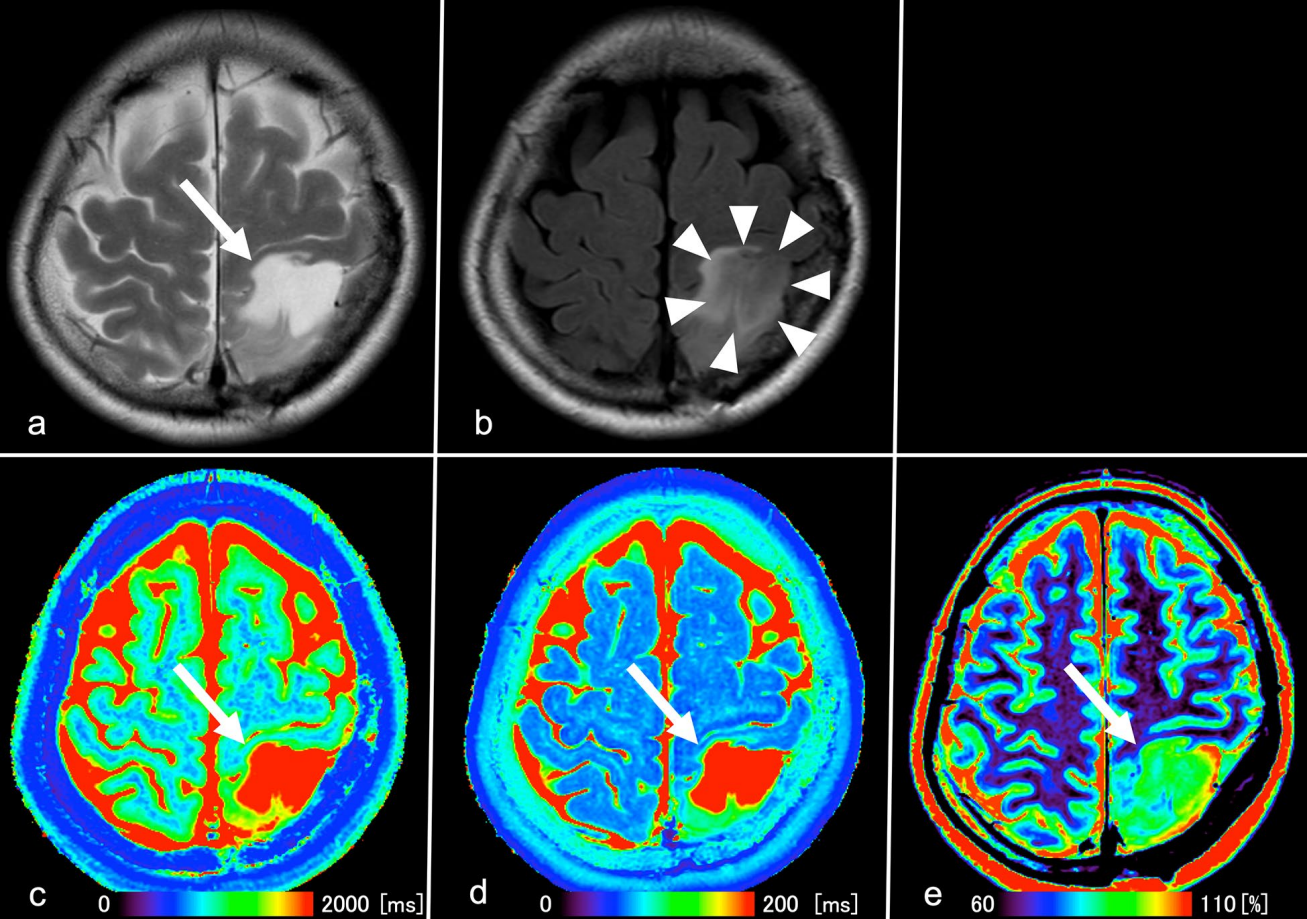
Images from a 49-year-old woman with IDH-mutant diffuse astrocytoma (WHO grade II). (a) T2WI shows a heterogeneous T2-prolonged mass in the left parietal lobe (arrow). (b) FLAIR shows partial signal suppression, indicating a T2-FLAIR mismatch sign (arrowheads). (c), (d), (e), T1 and T2 relaxation time and proton density (PD) maps derived from SyMRI show T1 (2436 ms*) and T2 (287 ms*) relaxation time prolongations and increased PD (94.9%*) (arrows) in the tumor. *Each value is expressed as the mean.
